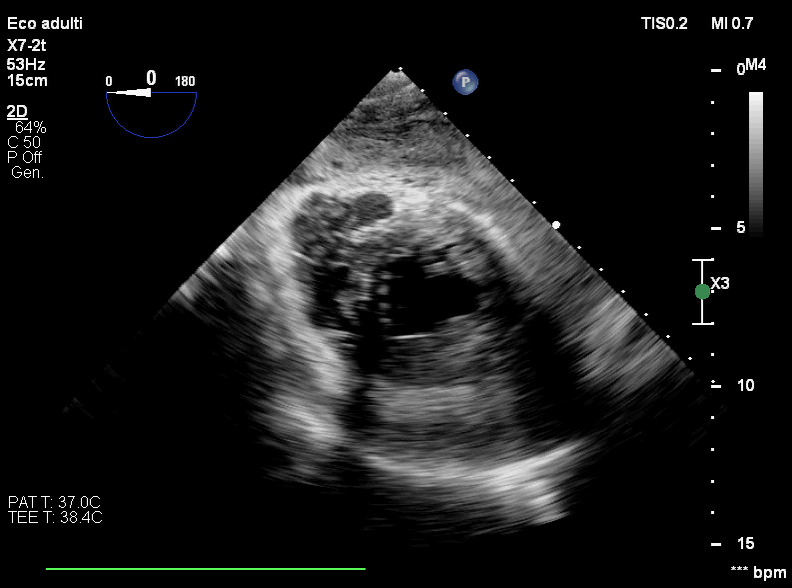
Transesophageal echocardiography (transgastric short-axis view) showing pericardial effusion with suspected posterior intrapericardial clot. Transgastric mid–short-axis TEE image demonstrating pericardial fluid with echogenic material along the posterior pericardial space, suggestive of organized clot/loculated postoperative collection. TEE, transesophageal echocardiography.
